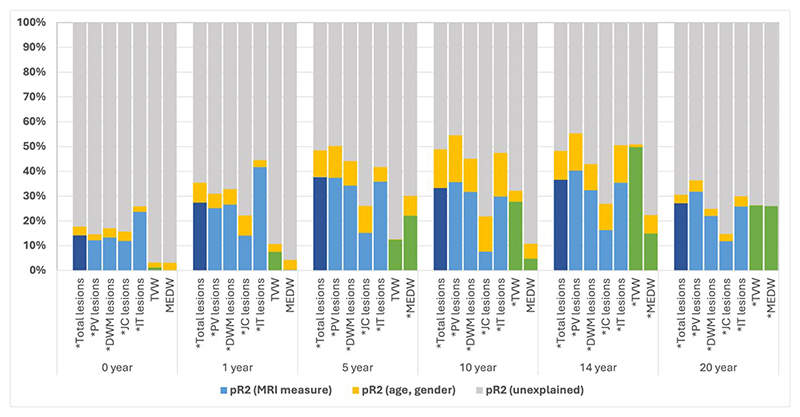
Cross-sectional models considering contribution of age, gender and MRI measures (total lesion count, lesion by subtype, linear atrophy measures) towards 30-year SPMS For all figures, * indicates a model that was significant at p≤0.05. At some timepoints age and gender explained small amounts of the outcome of interest and may therefore not be clearly seen in the Figures. Please see Tables 4 & 5 in supplementary materials for a detailed breakdown of the values.
